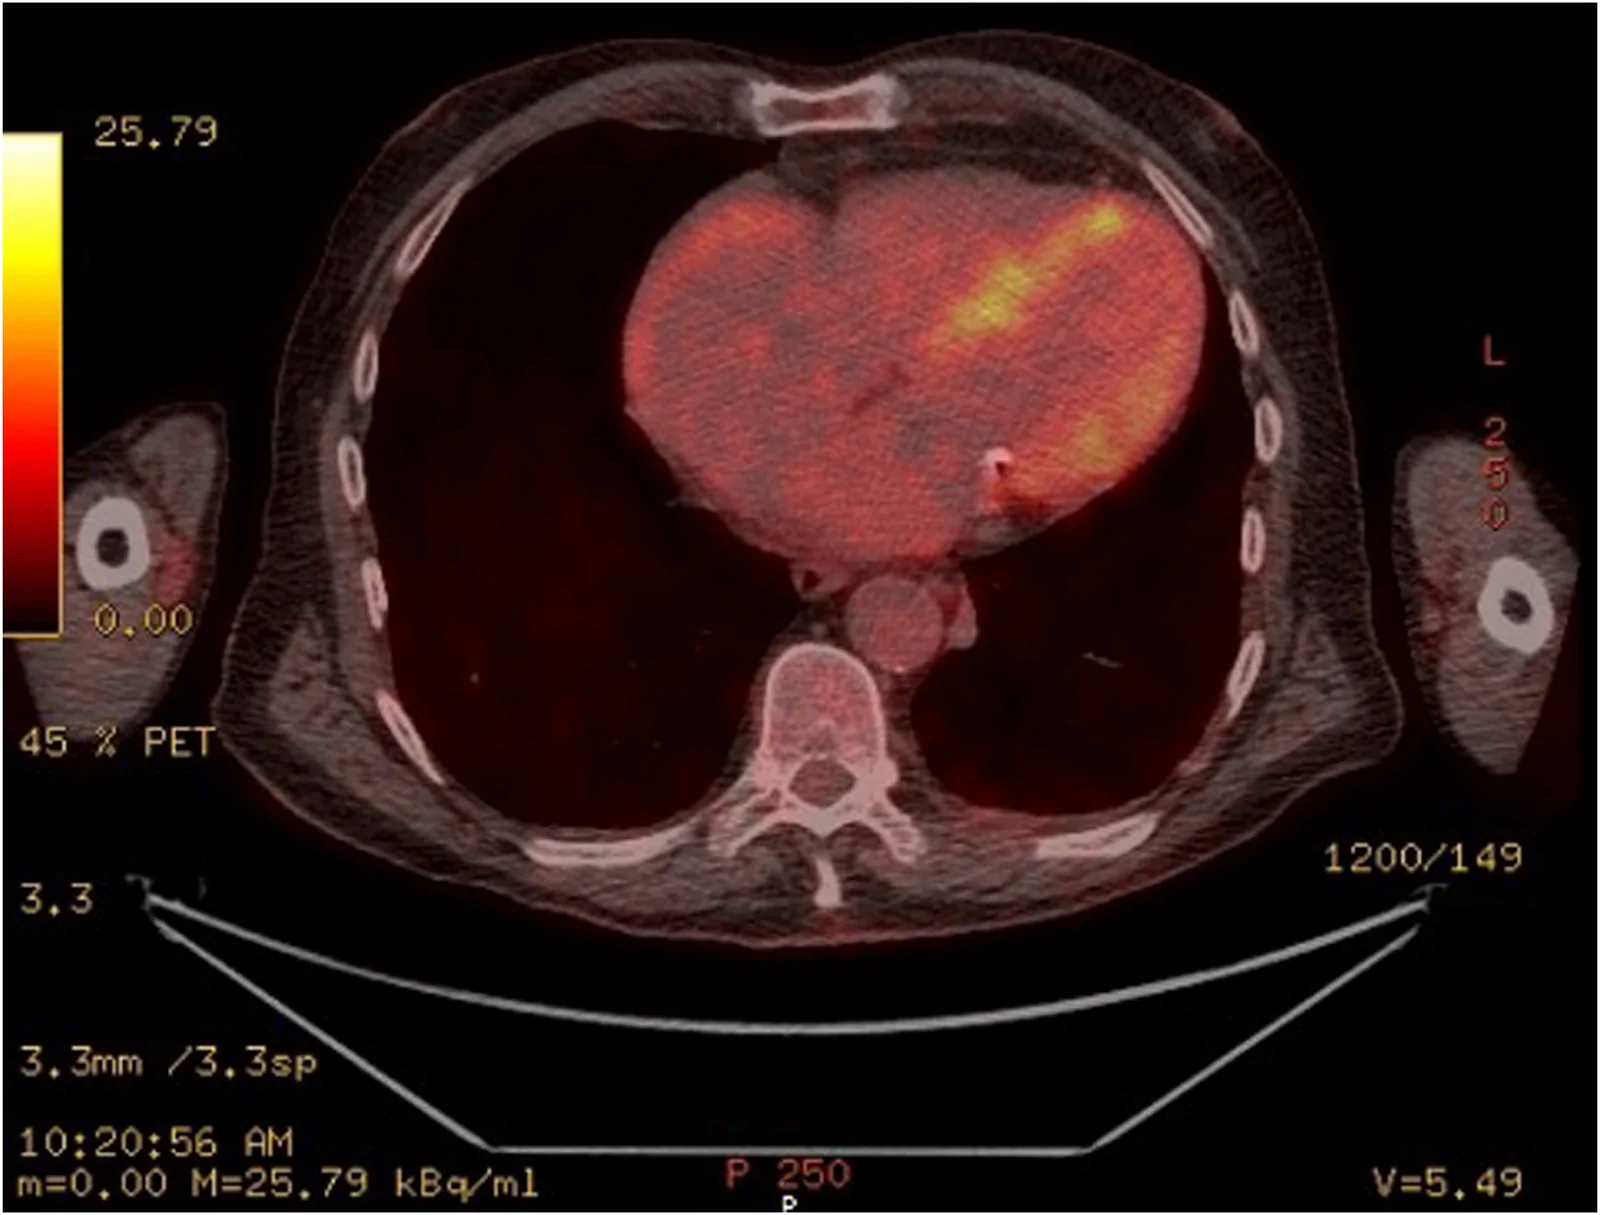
Cardiac PET/Computed Tomography Positron emission tomography (PET) scan shows heterogeneous fluorodeoxyglucose uptake in the left ventricular myocardium.
